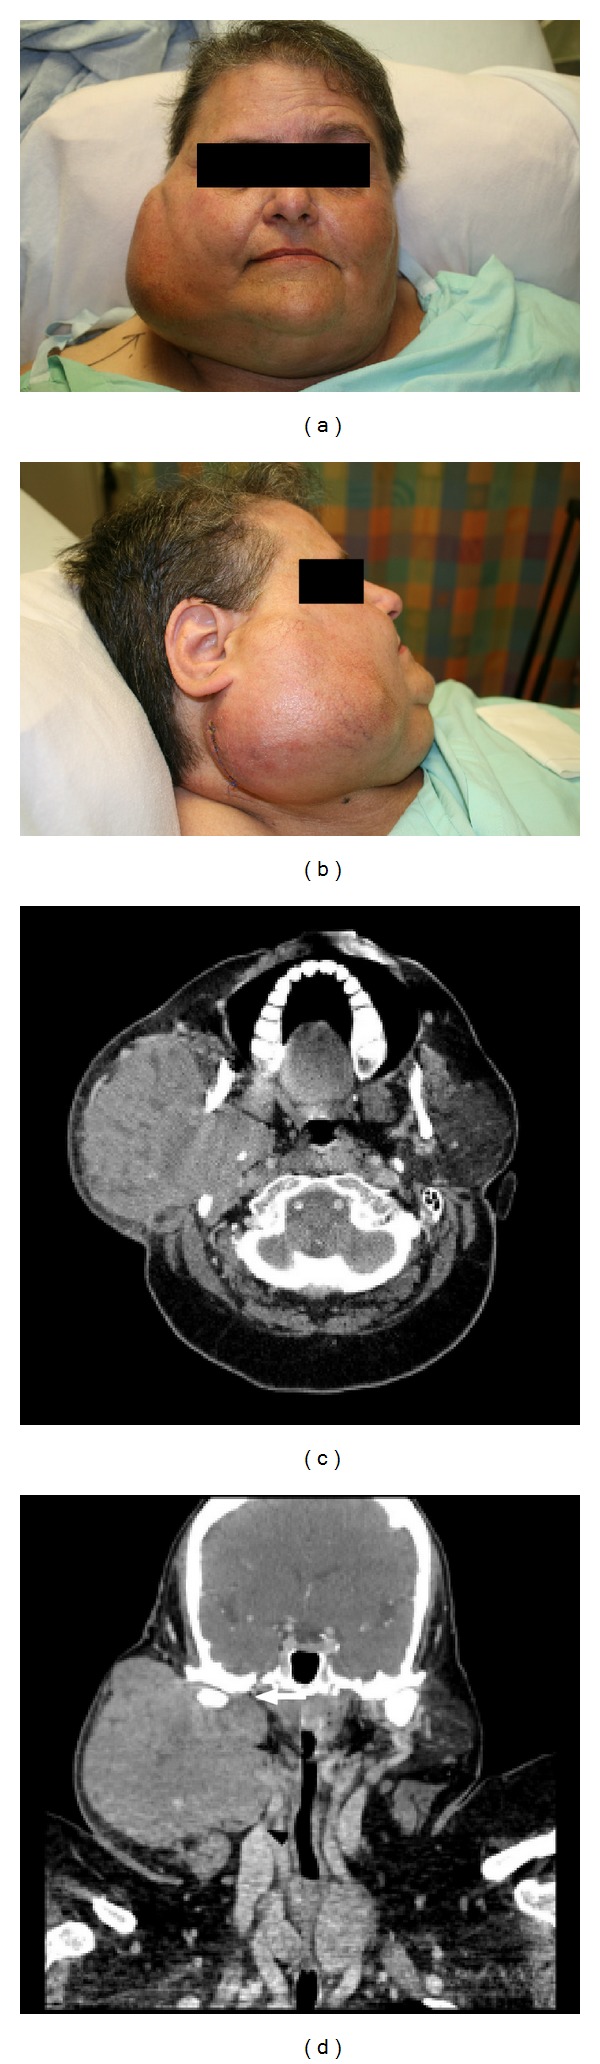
Anterior (a) and lateral (b) pictures of the patient at presentation. Axial (c) and coronal (d) CT scan images of the primary tumor. Note extension of the tumor into the parapharyngeal space with occlusion of the jugular vein (black arrowhead) and skull base involvement (white arrowhead).
